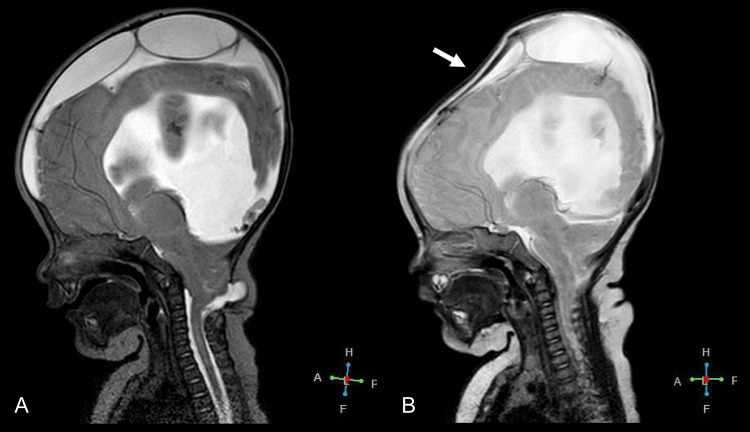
Comparison of MRI T2 sagittal images of the head before and after the subdural-peritoneal shunting procedure A) MRI before the subdural-peritoneal shunting; B) MRI after the subdural-peritoneal shunting, the white arrow points to the shrinkage of the external hydrocephalus MRI, magnetic resonance imaging
